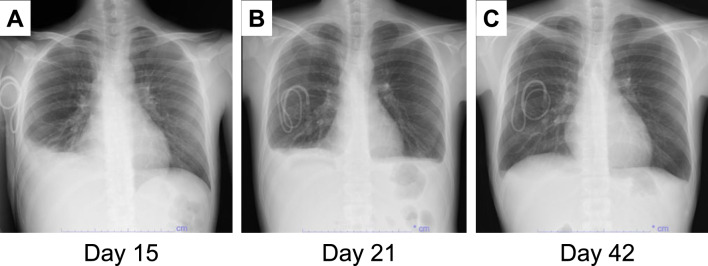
Changes in chest radiographic findings. Chest radiographs on day 15 (A), day 21 (B) and day 42 (C) after uPBSCT are shown
